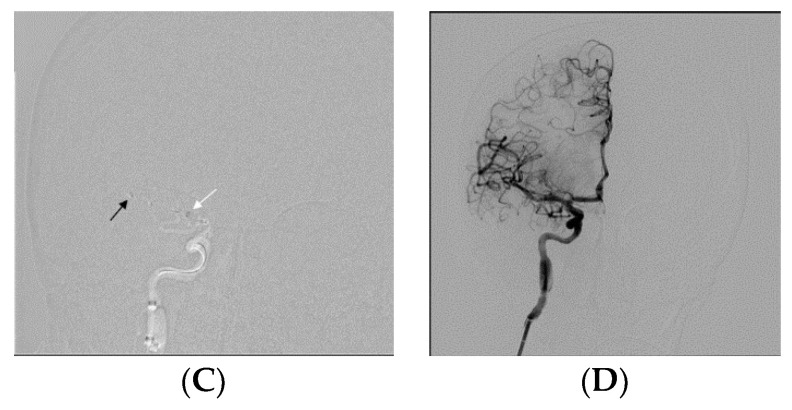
(A) Preprocedure CTA of the head indicated that the terminal end of the right ICA was occluded (black arrow) (T occlusion) (B) DSA of the right CCA confirmed the distal ICA occlusion (white arrow). (C) After the occlusion site was passed with a microcatheter, the stent retriever (black arrow) is completely deployed. 5F Catalyst catheter was connected to a Penumbra pump and was placed beyond the face of the thrombus (white arrow) via roadmap imaging. A balloon guide catheter was inserted into the proximal part of the petrous segment of ICA. (D) ICA and MCA were both recanalized with TICI 3 after a 1-pass.
